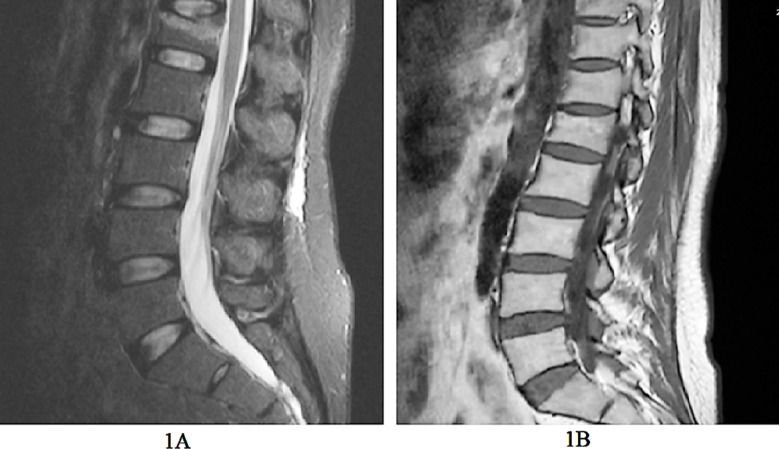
Representative magnetic resonance images of the lumbar spine of a patient with an osteoporotic fracture before and after treatment. 1A- MRI before treatment showing deformation of the first lumbar vertebral body wedge and patchy bone marrow edema; 1B- MRI after treatment showing a slightly flattened first lumbar vertebral body without bone marrow edema.
